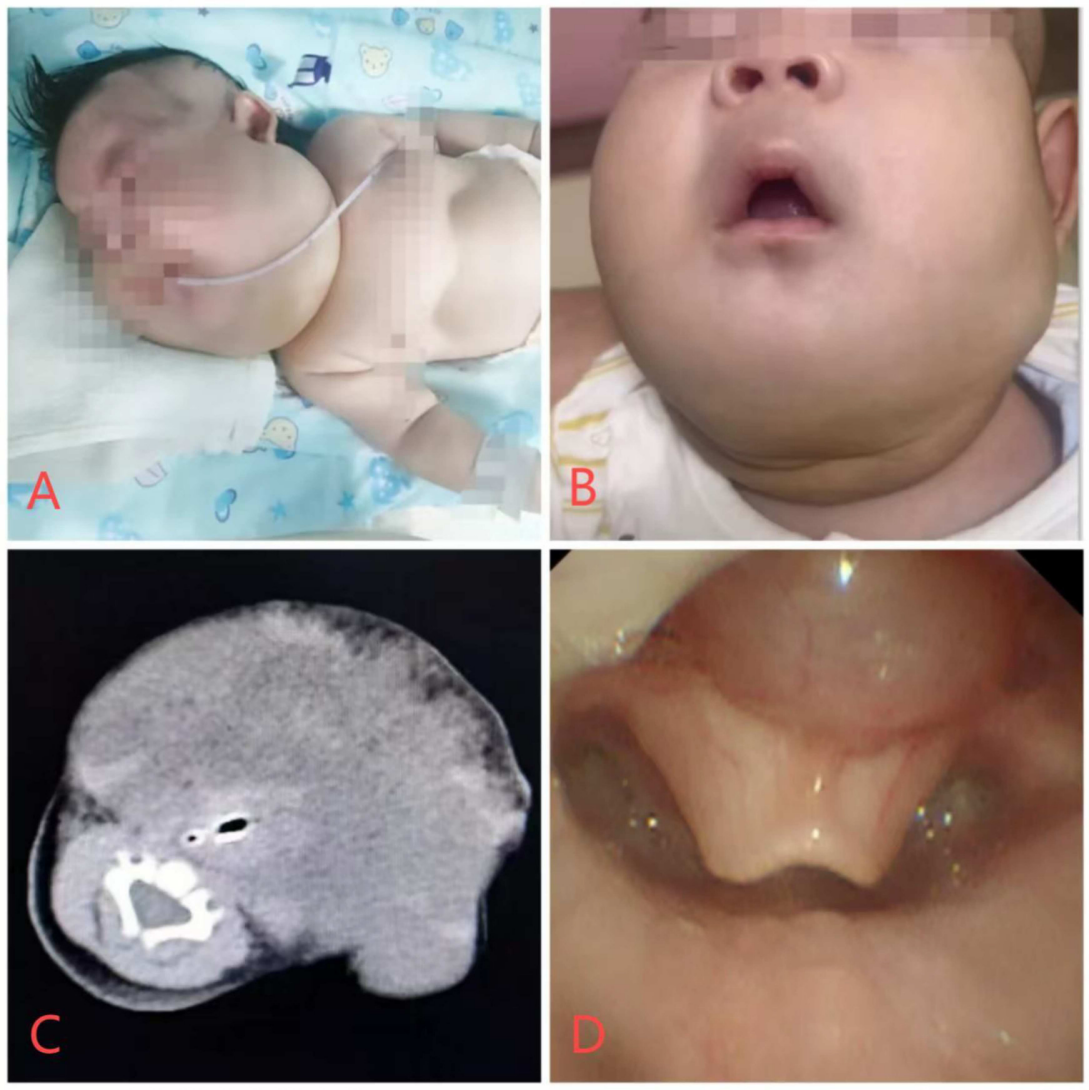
Patient photographs and imaging data. (A) Presence of suprasternal, supraclavicular, and intercostal retractions upon admission. (B) The patient's 8-month-old cervical lymphatic malformation has significantly reduced in size. (C) Cervical and Head CT Image of the Patient. (D) Bronchoscopic view of an epiglottic cyst.
